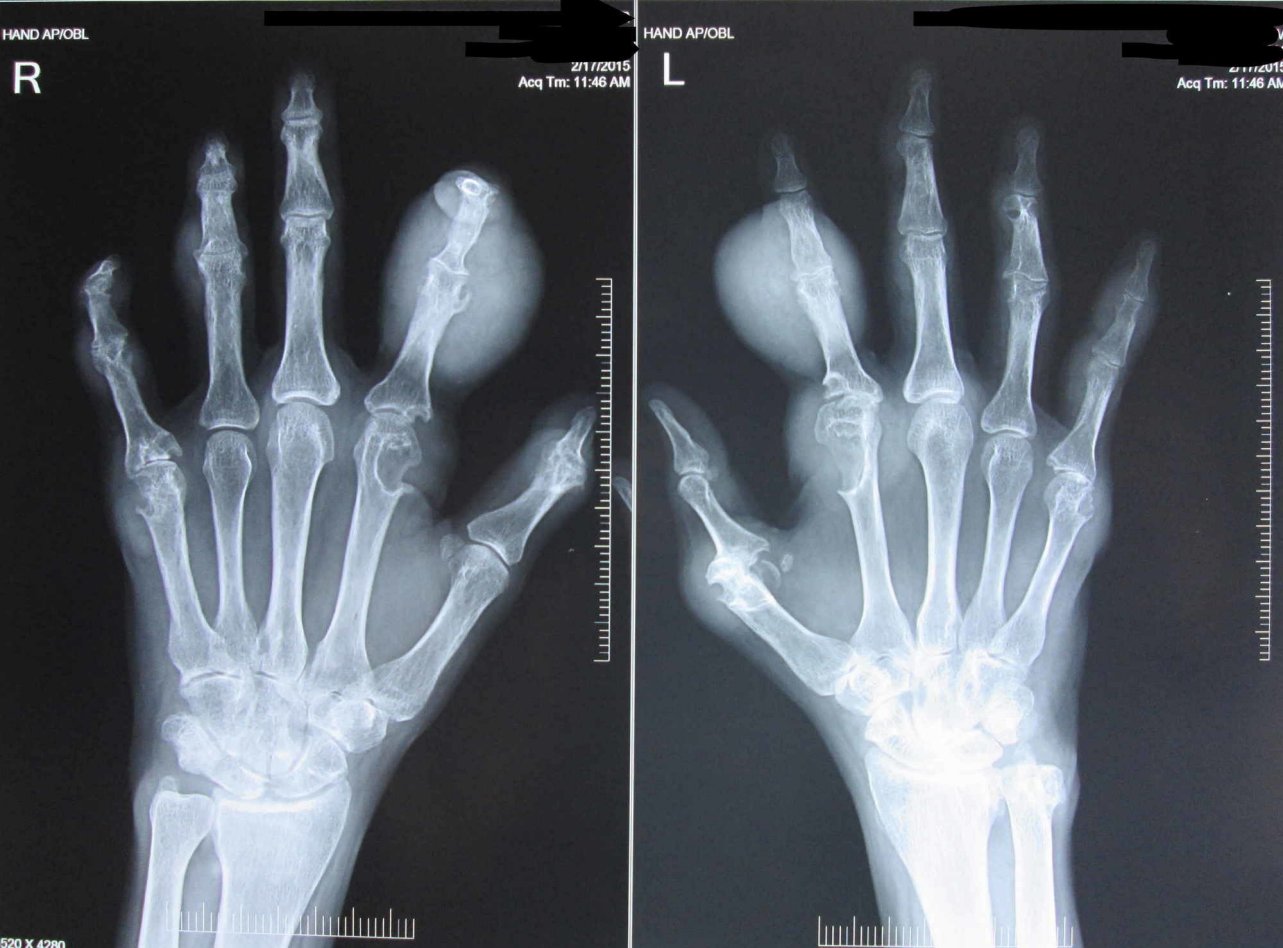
AP radiograph of bilateral hands showing soft tissue shadows in phalanges and periarticular punched-out erosions.
